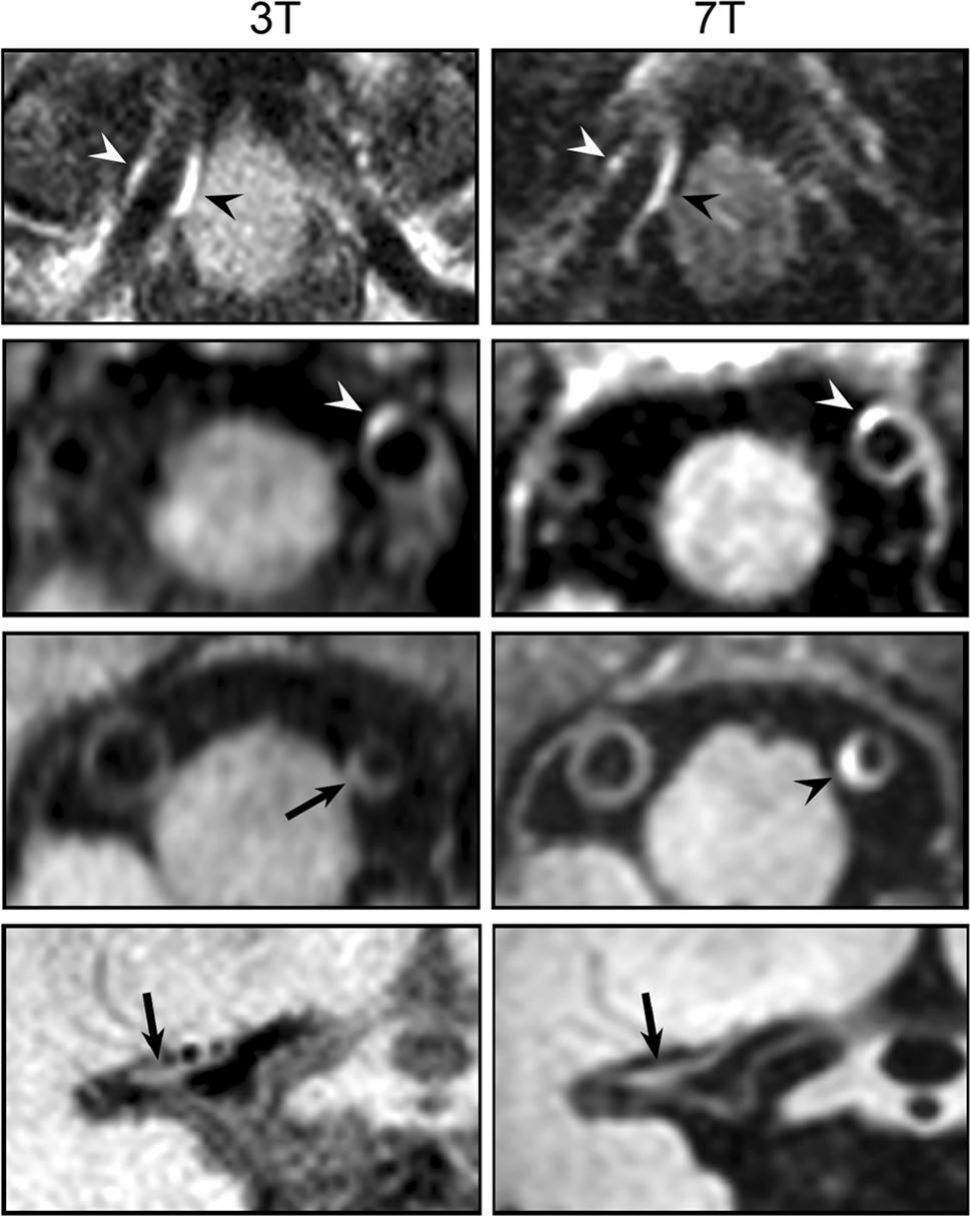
Comparison of post-contrast intracranial vessel wall imaging using 3T and 7T MRI. 7T MRI largely resulted in enhanced vessel wall delineation (due to enhanced CSF suppression) and more frequent detection of atherosclerotic lesions that were missed at 3T (*rows 3 and 4*). Depicted are the right proximal artery (*row 1*), left proximal vertebral artery (*rows 2 and 3*) and M1 segment of the middle cerebral artery (*row 4*). Adapted from Harteveld et al. [18]
